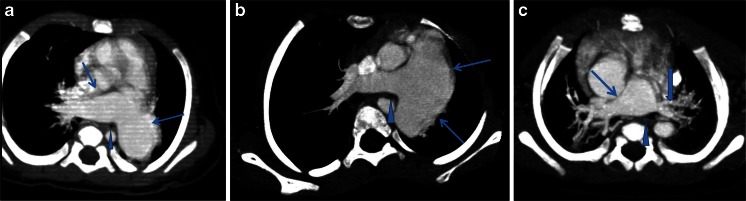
Multi-slice CT findings of absent pulmonary valve with or without ventricular septal defect and compressed bronchus. a Tetralogy of Fallot in a 4-month-old girl. Multi-slice CT axial-oblique maximum-intensity projection reconstruction demonstrates right and left pulmonary artery dilatation (*arrows*). Right and left main bronchi are inferior and posterior to the dilated right pulmonary artery. The left bronchus looks displaced posteriorly and compressed (*arrowhead*). b Absent pulmonary valve, intact ventricular septum and atrial septal defect in a 17-month-old girl. Multi-slice CT axial-oblique maximum-intensity projection reconstruction demonstrates main pulmonary artery and left pulmonary artery dilatation (*arrows*), which is markedly compressing the left bronchus (*arrowhead*). c Absent pulmonary valve syndrome with double-outlet right ventricle, ventricular septal defect, atrial septal defect and left superior vena cava in a 3-month-old boy. Multi-slice CT axial maximum-intensity projection reconstruction demonstrates a dilated right pulmonary artery (*thin arrow*) and hypoplastic left pulmonary artery (*thick arrow*). The proximal left bronchus looks compressed by the dilated right pulmonary artery (*arrowhead*)
